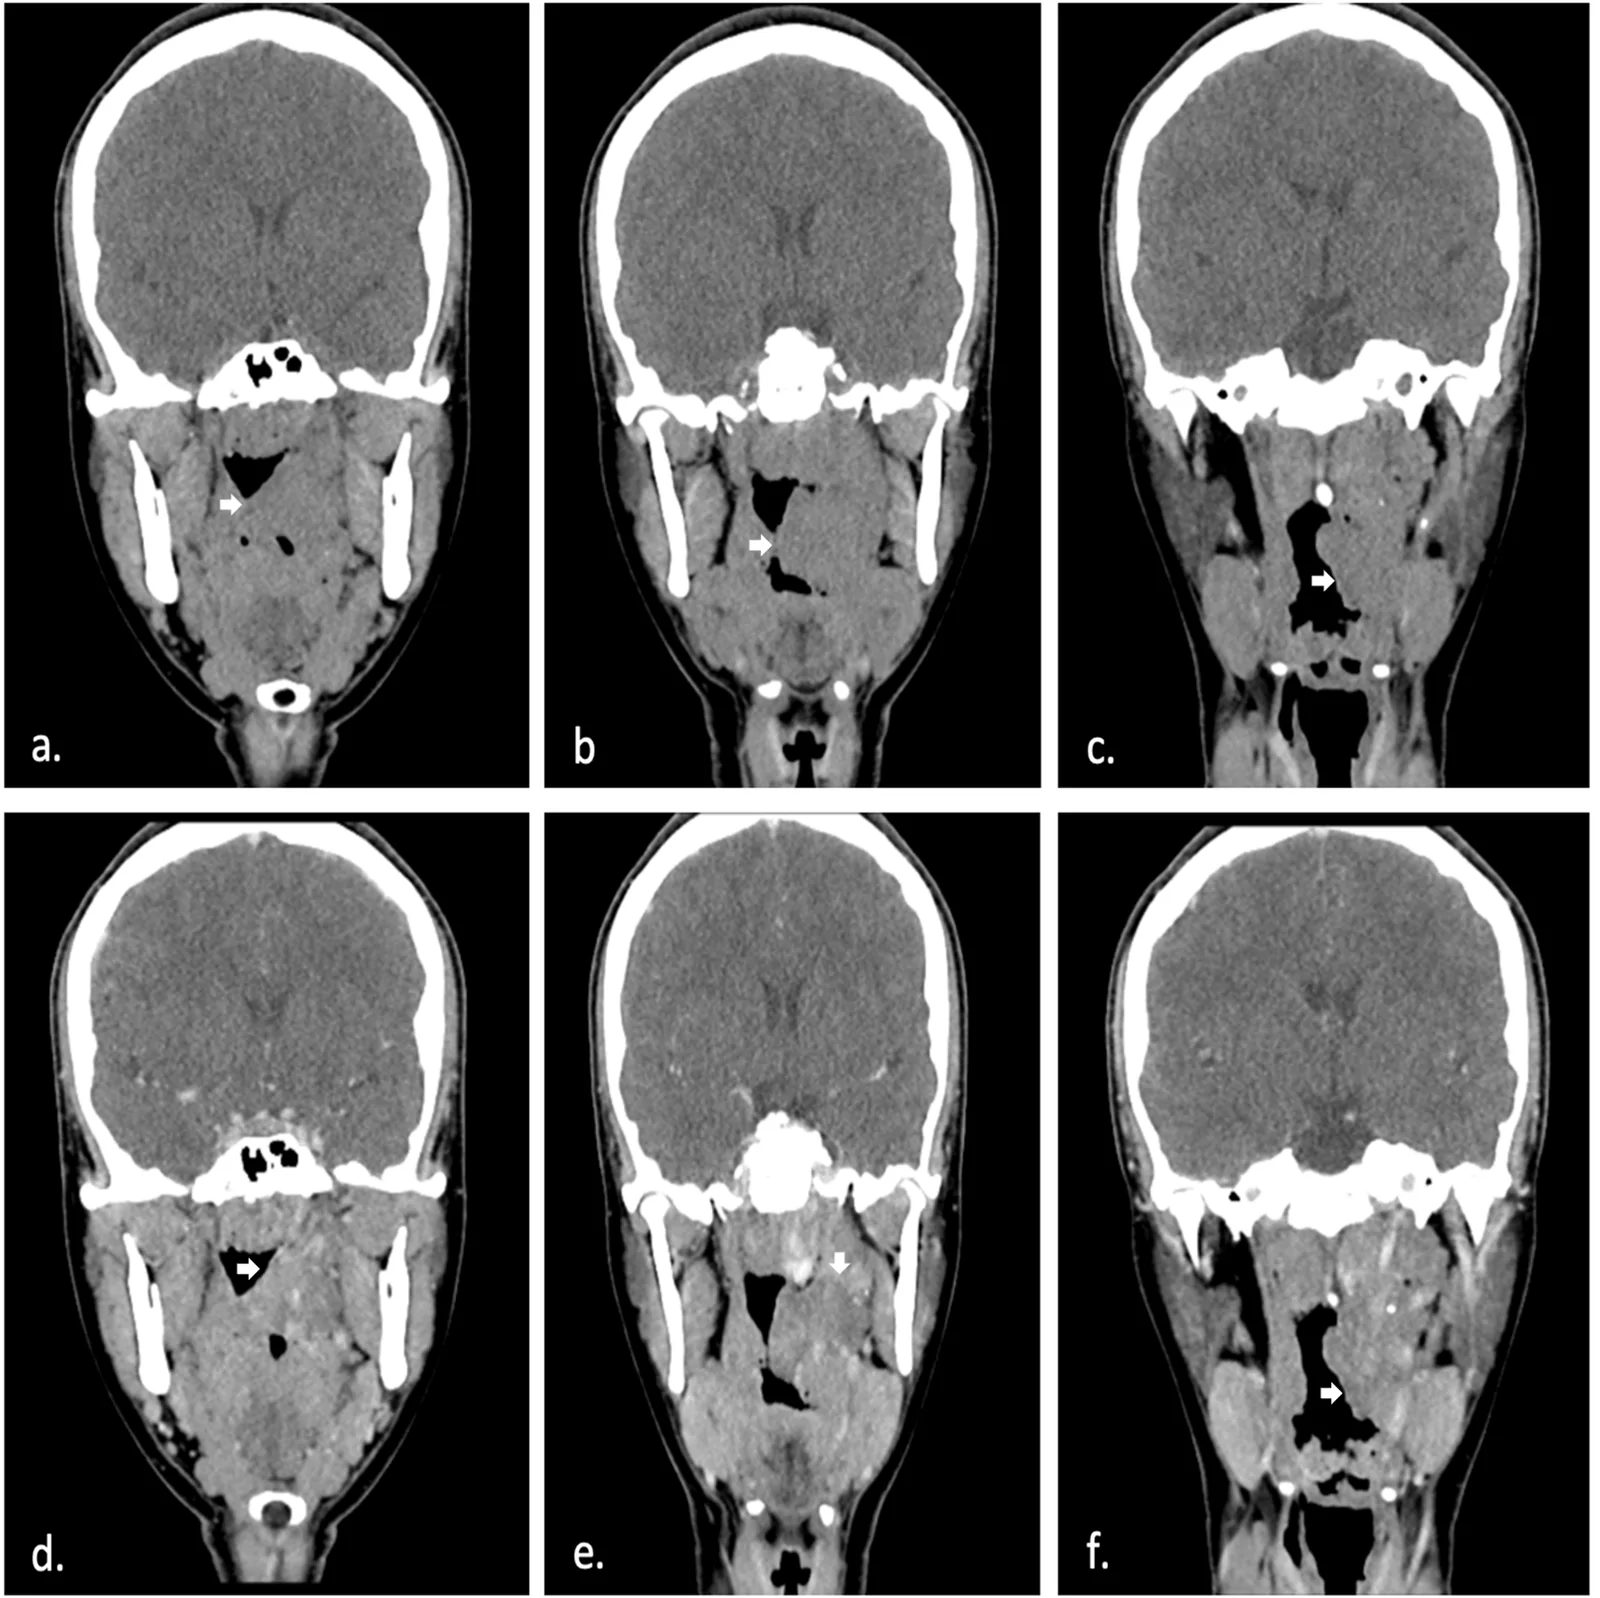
CT angiography Coronal non-contrast (a–c) and contrast-enhanced (d–f) CT angiographic images demonstrate an extensive hypervascular soft-tissue mass centered in the left soft palate, with superior extension into the nasopharynx and inferior extension into the oropharynx. Following intravenous contrast administration, the lesion exhibits heterogeneous enhancement, allowing precise delineation of its anatomical extent and vascular characteristics.
